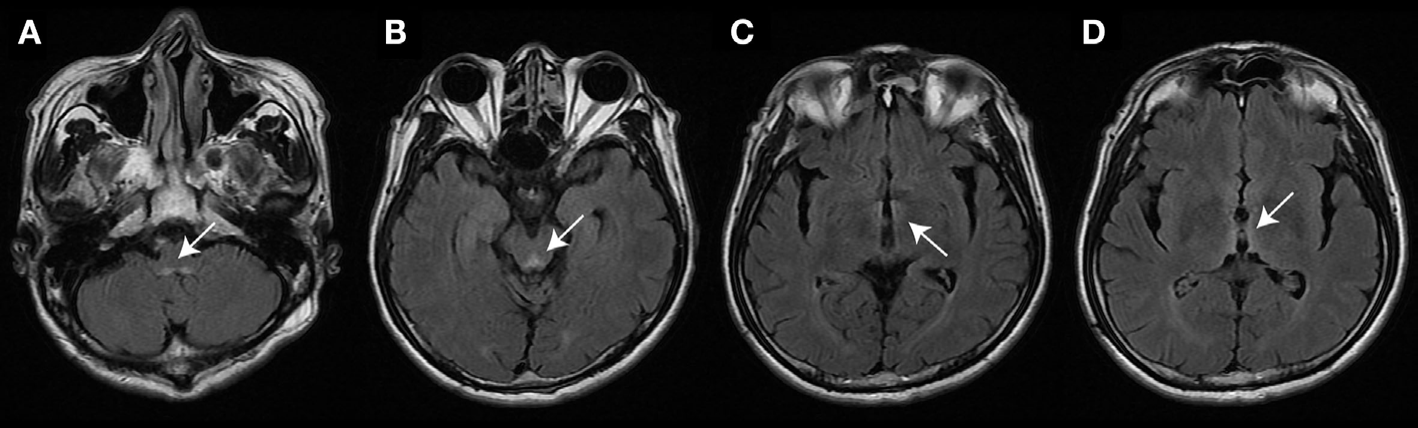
Brain magnetic resonance image (patient #1) studies (fluid-attenuated inversion recovery images) show bilateral symmetrical lesions (as marked by white arrows) in the medial vestibular nucleus (A), the periaqueductal region (B), around the hypothalamus and mammillary bodies (C), and the periventricular regions of the thalamus (D).
